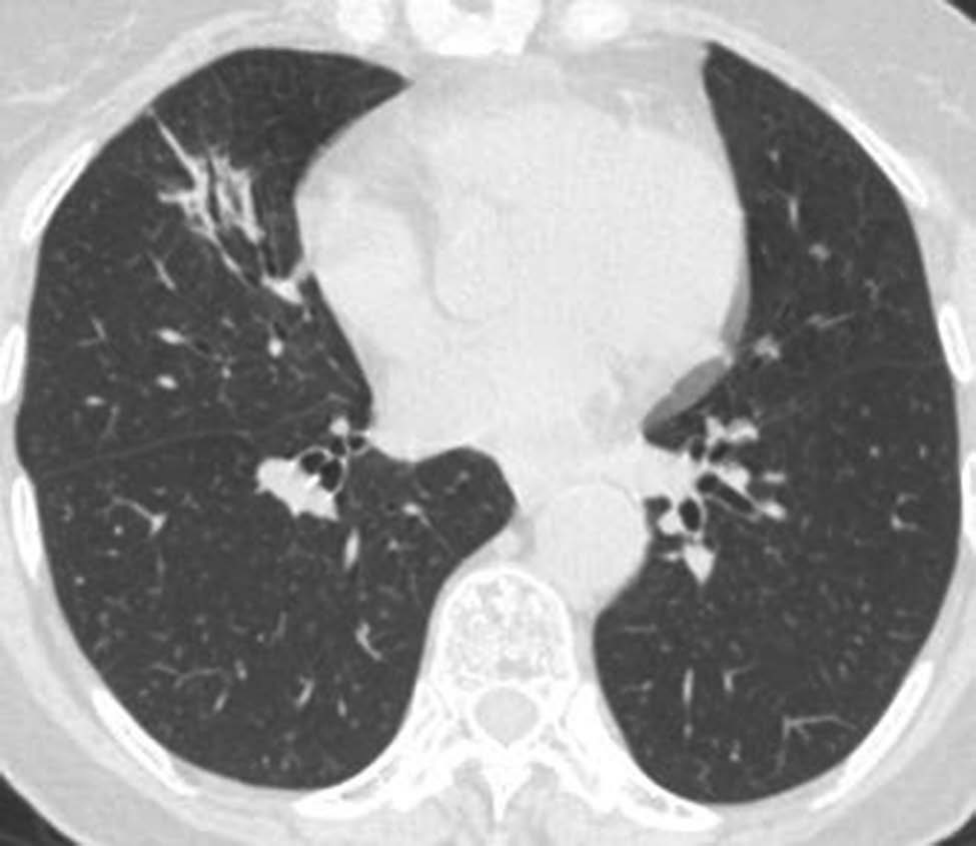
Female, 65 years old. Chest CT showed mixed ground glass nodules in the middle lobe of the right lung, and the maximum section diameter was about 24 mm * 21 mm, circular, clear boundary, marginal lobation, uneven lesion density, tortuous and thickened vessels and inflatable bronchi.
